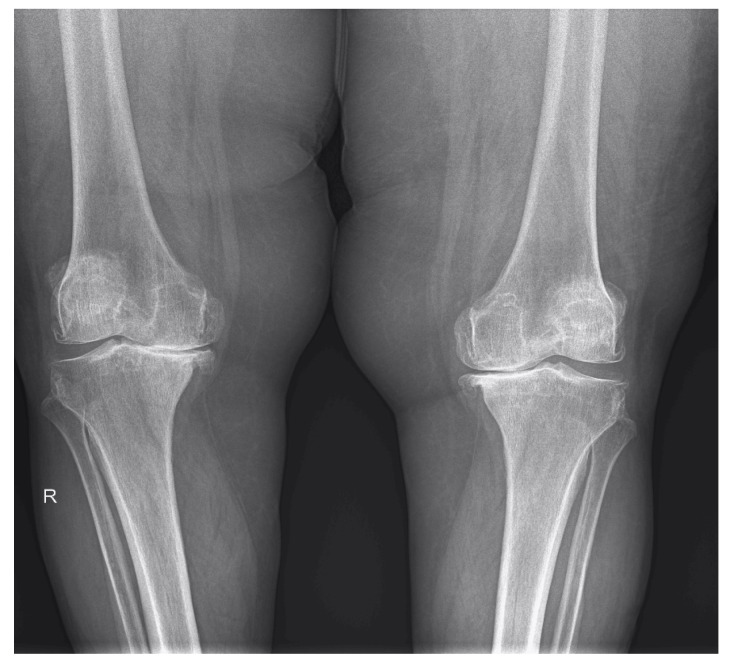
Radiograph of the knees in anterior view showing marked reduction of the joint space, predominantly in the medial compartment, subchondral sclerosis of the tibial plateau, and medial and lateral femoral and tibial osteophytes. R—right.
